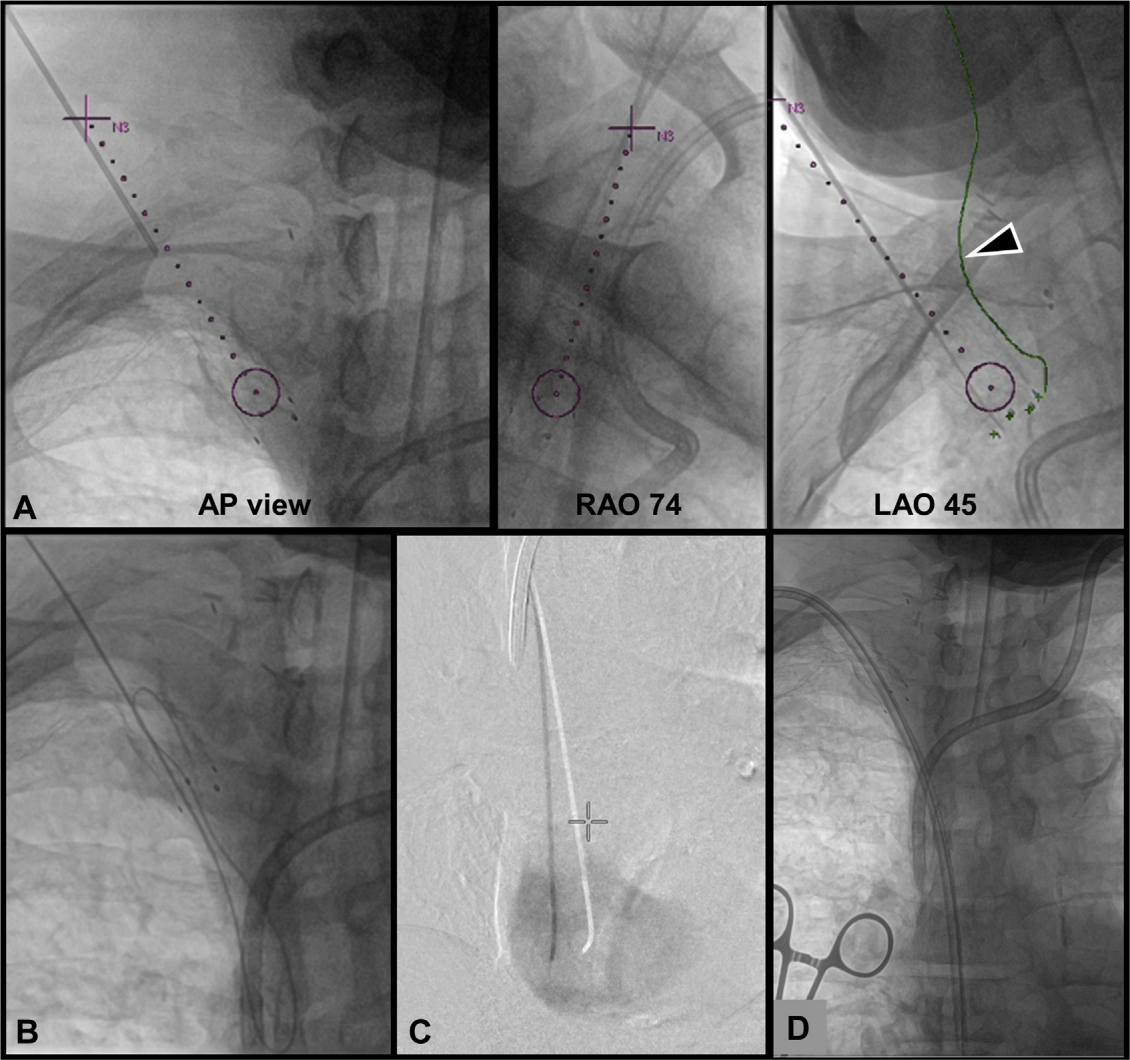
Intraoperative Cone-Beam Computed Tomography and Fluoroscopy Image Guidance for Percutaneous Recanalization of the Occluded Stent (A) Overlay of a 3-dimensional virtual path from cone-beam computed tomography onto 2-dimensional fluoroscopy for guiding percutaneous recanalization of an occluded stent (purple dotted line); the green line indicates the lateral margin of the right common carotid artery. (B) Puncture and wire passage into the superior vena cava (SVC) with (C) confirmation of catheter position in the right atrium. (D) Placement of a tunneled dialysis catheter. AP = anteroposterior; LAO = left anterior oblique; RAO = right anterior oblique.
